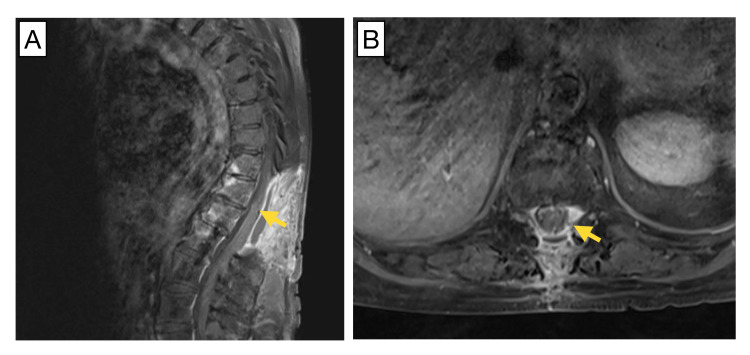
Postoperative MRI of the spinal column. Sagittal (A) and transverse (B) postoperative MRI demonstrate excision of the left lateral mass (yellow arrows).
